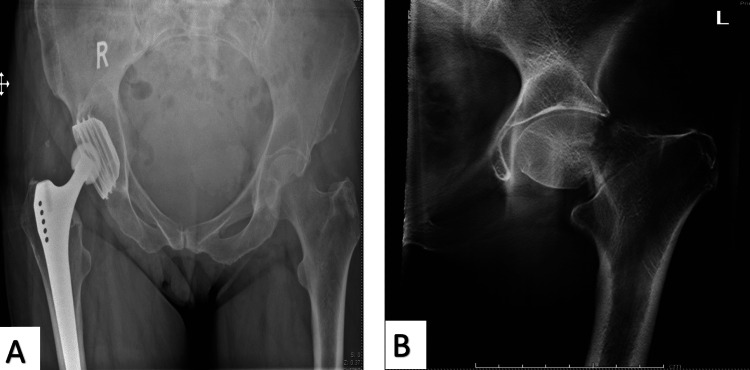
A 79-year-old woman missed a step and presented with left hip pain and struggling to bear weight. The plain radiograph did not show fracture (A), but the tomogram showed a minimally displaced femoral neck fracture (B).
